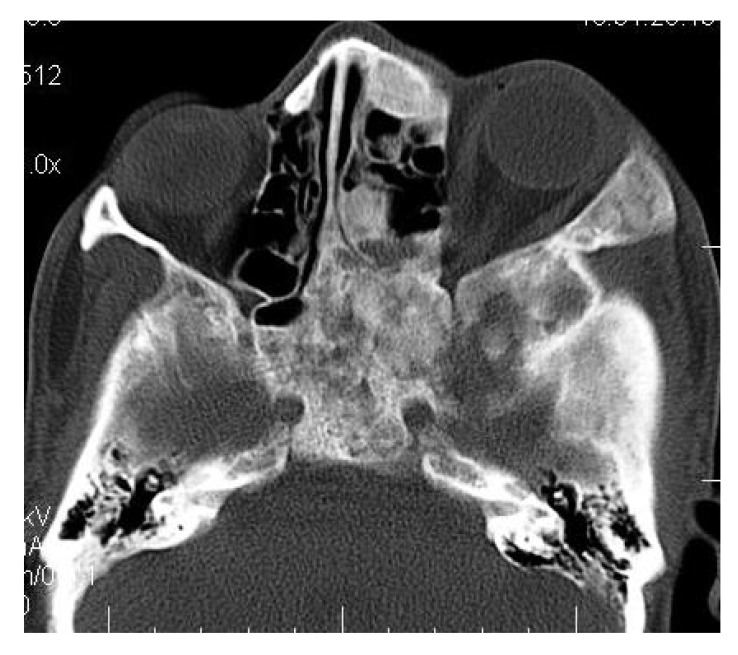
Axial CT slice with bone window revealing involvement of temporal, zygomatic, frontal and ethmoid bones. Sphenoid bone was also involved with narrowing of the optic canal as well as orbital fissures.
